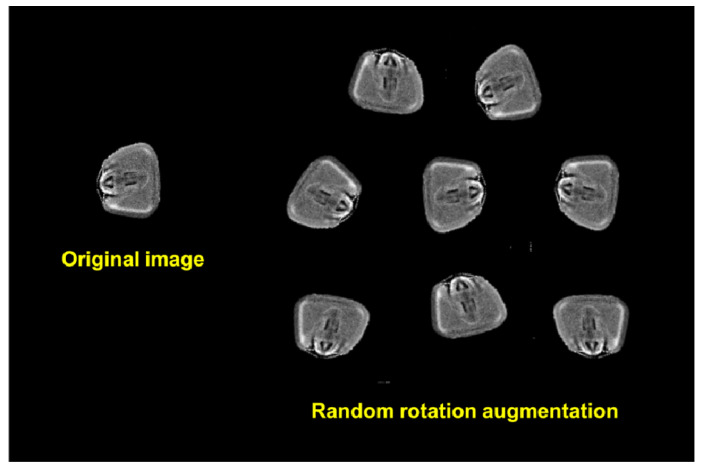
Data augmentation method based on random rotations and flips (mirror effect) used for X-ray image recognition in classes of maize grains infested and non-infested by *Sitophilus zeamais*.
